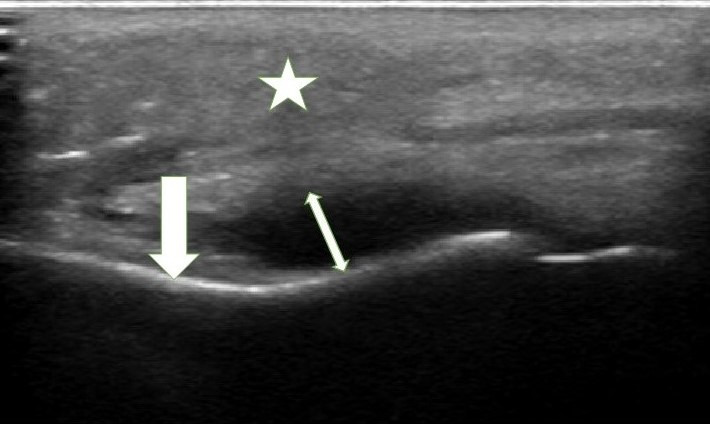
Point of care ultrasound (POCUS) image of dental abscess. Star indicates buccal soft tissue. Single arrow indicates maxillary cortex. Double-headed arrow demarcates the maximum height of an anechoic dental abscess.
